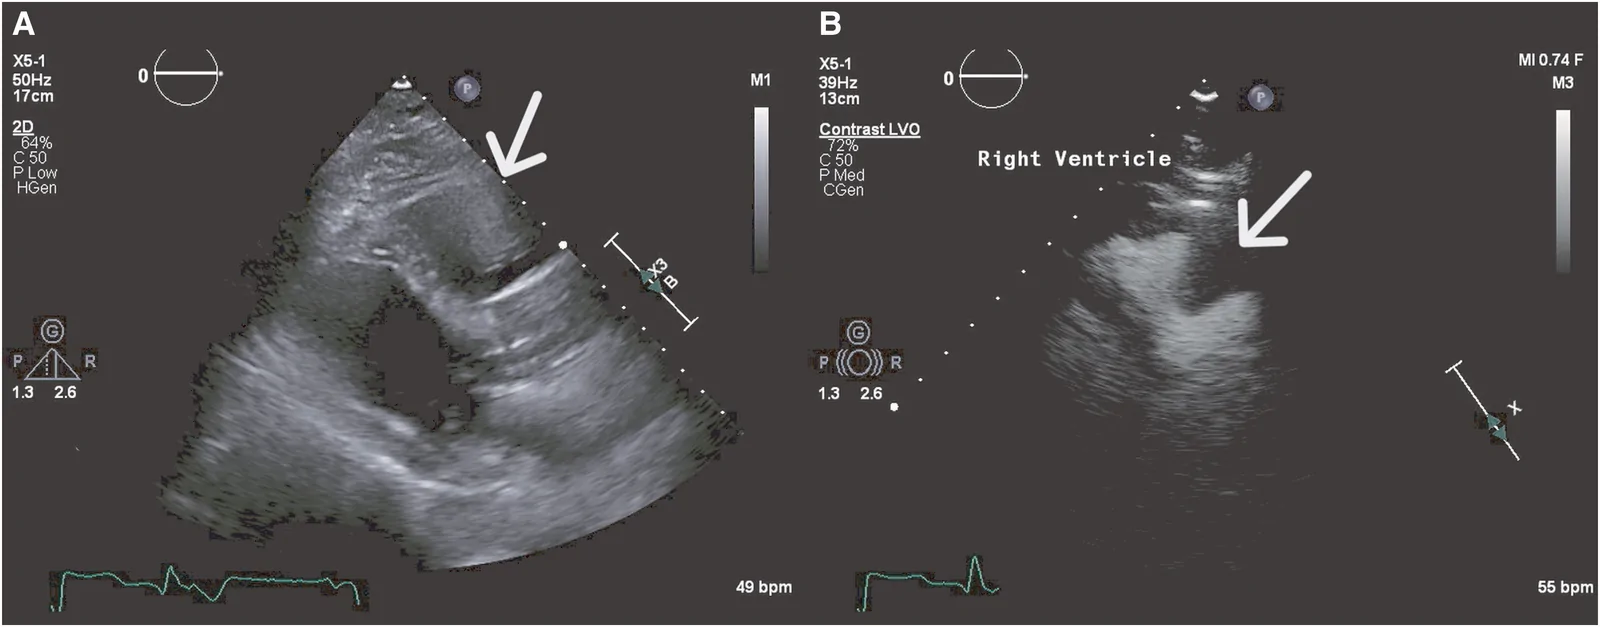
Transthoracic echocardiogram parasternal view. (*A*) Demonstration of the right ventricular mass measuring ∼2.4 × 2.9 cm, described as a wedge-shaped, broad-based, slightly hypoechoic mass involving the right ventricle and right ventricular outflow tract (indicated by white arrow). (*B*) Contrast-enhanced imaging demonstrates no contrast uptake within the mass (indicated by white arrow).
